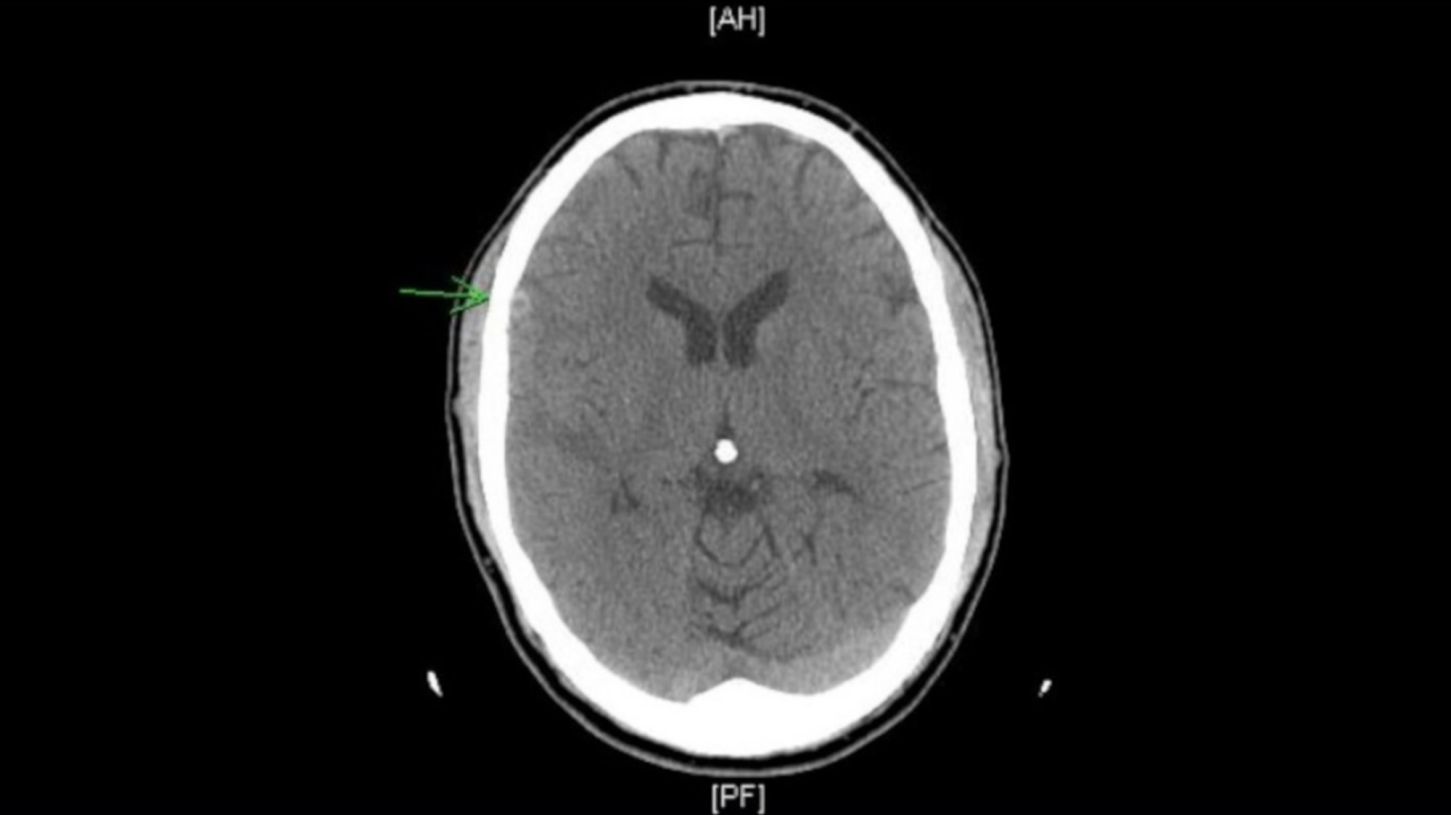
Noncontrast computed tomography (CT) of the head showing increasing cerebral edema on the right parietal lobe with no increasing mass effect or midline shift.
